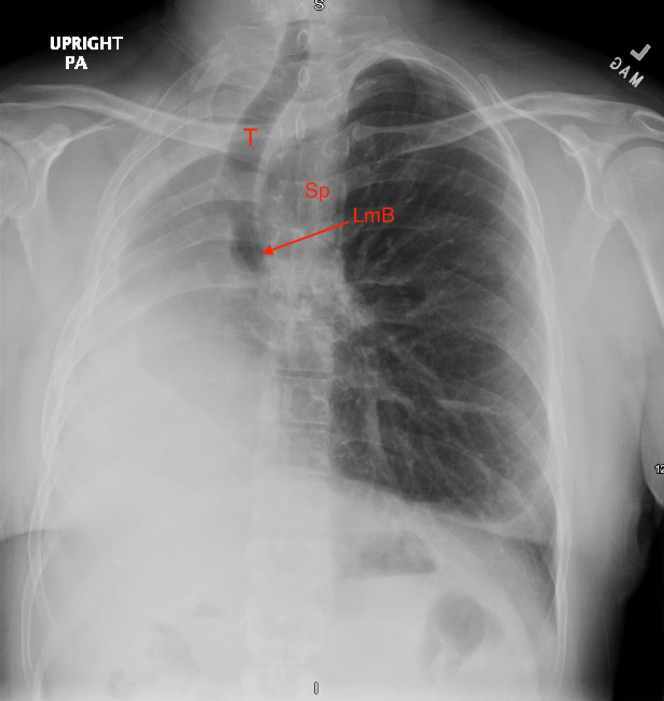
Chest Radiograph Depicting Complete Mediastinal Shifting in Postpneumonectomy Syndrome Anterior-posterior chest radiograph of a hyperinflated left lung with complete deviation of mediastinal contents including rightward shift of the trachea (T) and stretch of the left main bronchus (LmB, red arrow), past the vertebral column/spine (Sp).
